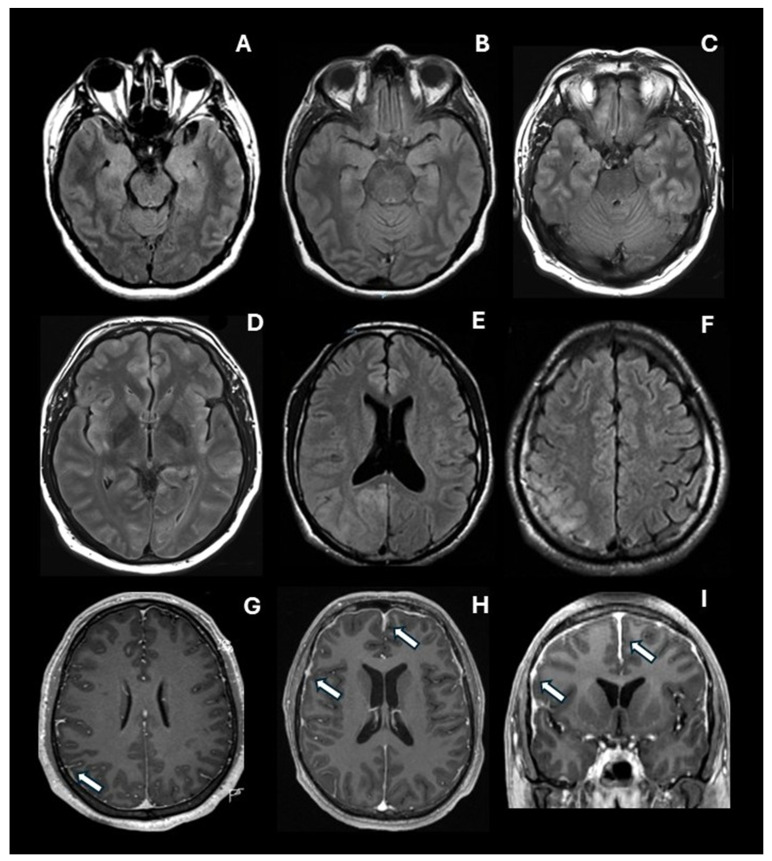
Brain MRI abnormalities in anti-NMDA receptor encephalitis. Limbic, paralimbic, and neocortical abnormalities found in the T2 T2-FLAIR sequence are depicted. Bilateral hyperintense signal can be seen: (A) in the uncus and the amygdala, bilaterally; (B) in the hippocampus, bilaterally; (C) in the medial aspects of the temporal lobe, bilaterally, and in the left lateral temporal cortex; (D) in the insular cortex, and the anterior cingulate cortex, bilaterally; (E) in the right anterior cingulate cortex and retrosplenial cortex; and (F) in the right lateral parietal cortex. Also, representative images of contrasted T1 sequence with leptomeningeal (G) and pachymeningeal (H,I) enhancement are shown and highlighted through the arrows.
